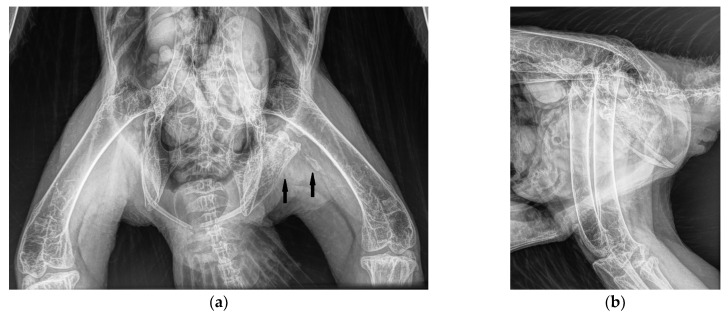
Ventro-dorsal (a) and latero-lateral (b) radiographs of a bald eagle (case 2) with heterotopic bone formations with a smooth surface between the femur and pelvis along the adductors (arrows).
